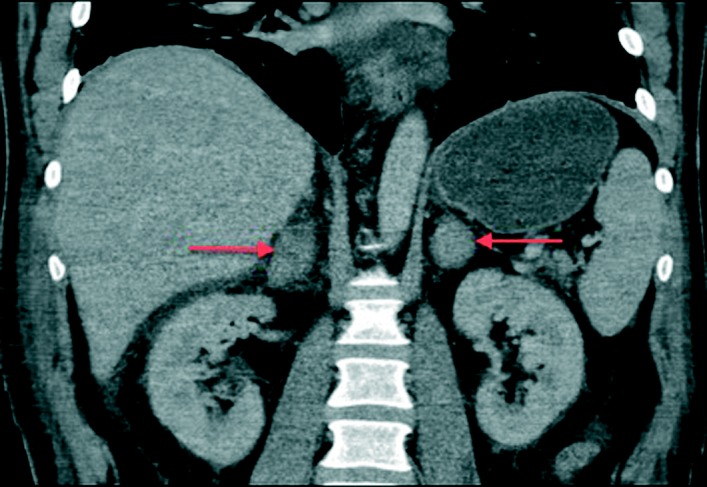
Coronal view of CT adrenals revealing haemorrhagic adrenal glands (arrows).
